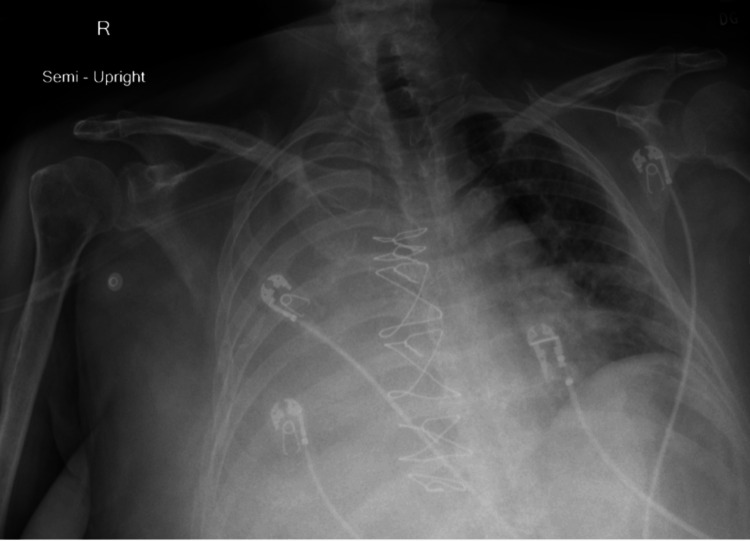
Chest radiograph of the patient The image shows complete opacification of right hemithorax, suggestive of large right pleural effusion
